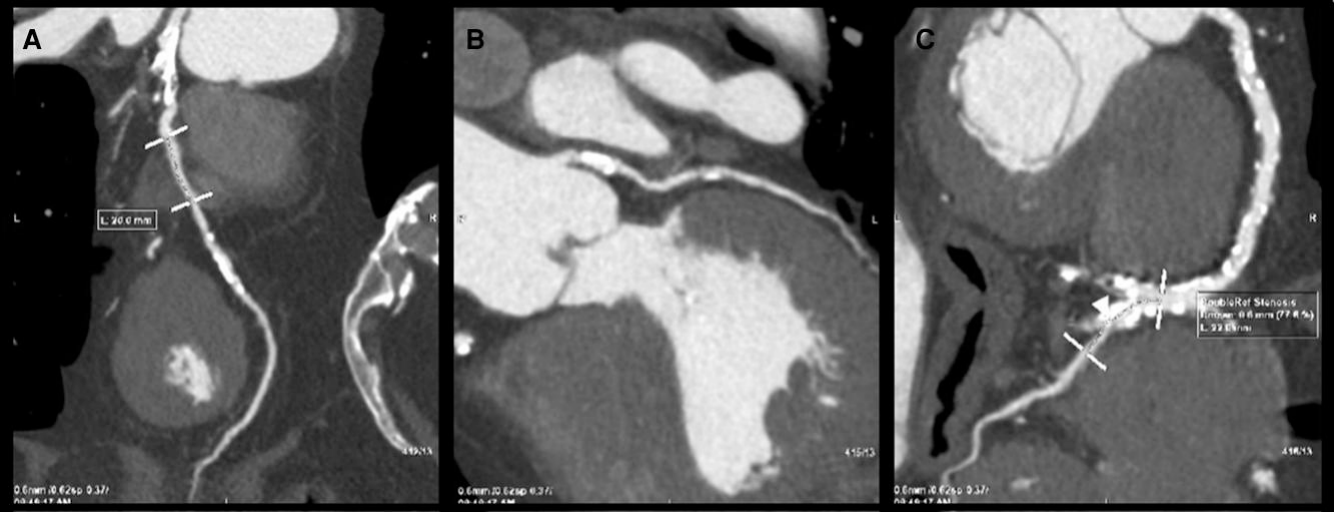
Computed tomography of critical coronary artery disease at pre-ablation scan. (*A*) Curved multiplanar (MPR) image of severe stenosis in proximal left anterior descending (LAD) caused by a calcified plaque. (*B*) Curved MPR image of severe stenosis in the proximal LCX caused by a calcified plaque. (*C*) Curved MPR image of a severe stenosis in the proximal RCA caused by both calcified and non-calcified plaques.
